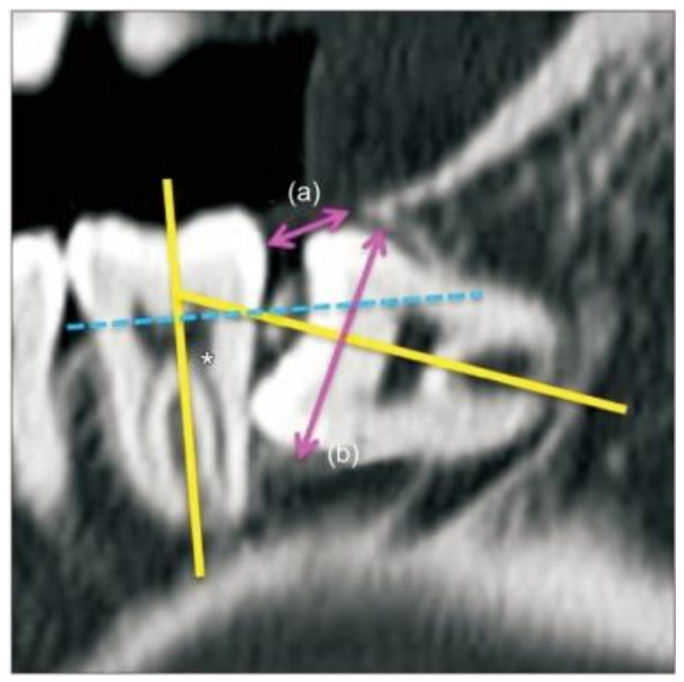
Measurement and classification of the impacted third molars in cone-beam computed tomography [12]. The spatial relationship was subcategorized based on the angle between the long axis of the third molar and the adjacent second molar as follows (yellow lines and an asterisk mark): (1) mesioangular (11° to 79°), (2) horizontal (80° to 100°), (3) vertical (−10° to 10°), (4) distoangular (−11° to −79°), or (5) reverse, where the crown of the third molar was more root-oriented than horizontal. The depth was classified based on the line connecting the cementoenamel junction of the adjacent second molar (dotted blue line) as follows: (1) more than half of the third molar crown was above the CEJ of the adjacent second molar; (2) less than half of the third molar crown was above the CEJ of the adjacent second molar; (3) more than half of the third molar crown positioned superior to the mid-level of the adjacent second molar root; (4) the third molar crown level inferior to that mentioned above. The ramus relationship/space available was subcategorized based on the ratio between the distance from the ascending ramus to the distal of the second molar (a) and the diameter of the impacted third molar (b) (pink arrows). An eruption space (a/b) larger than two-thirds of the distance was defined as (1), between one-third and two-thirds—as (2), and smaller than one-third—as (3).
